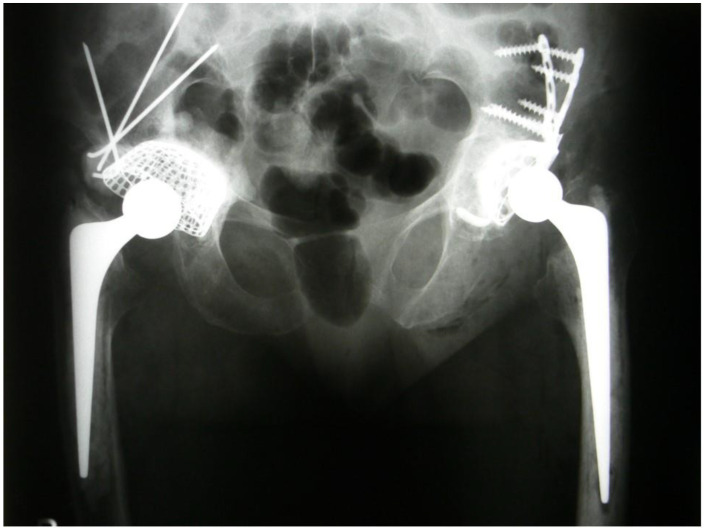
Status post revision arthroplasty of the right hip joint and primary arthroplasty of the left hip joint. An AP radiograph of the pelvis and the hip joints.
